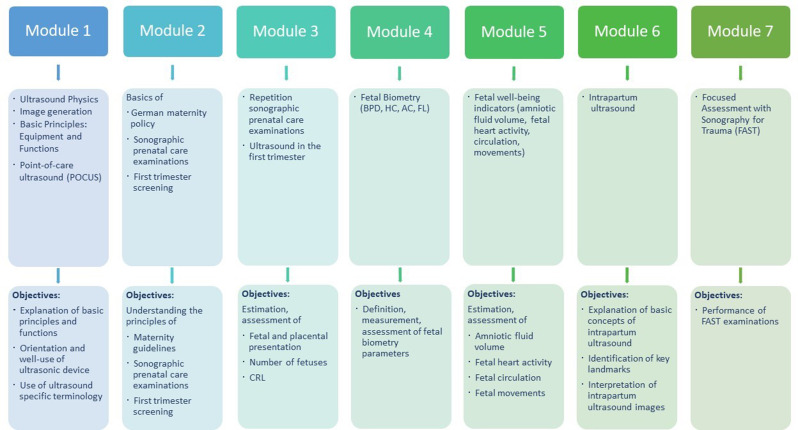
Modules and learning objectives of the obstetric ultrasound course. AC: abdominal circumference, BPD: biparietal diameter, CRL: crown rump length, FL: femur length, HC: head circumference
